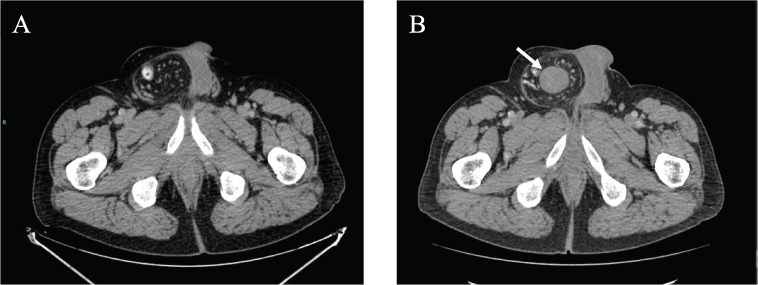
(a) September 2020 CT scan showing a right inguinal hernia. (b) September 2021 CT scan showing a solid nodular lesion of 26 × 40 × 75 mm inside the already-known inguinoscrotal hernia.
